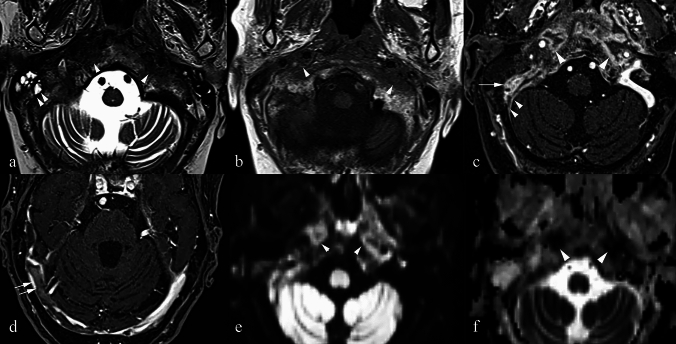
Elderly patient presenting with VII cranial nerve palsy and right-sided otalgia. a Axial T2 TSE at the level of the clivus and petrous apex demonstrates heterogeneous, low signal intensity changes throughout the bone marrow (arrowheads) associated with bilateral mastoiditis worse on the right (double arrowhead). b Axial T1 SE at the same level confirms the pathological marrow replacement c T1 VIBE post-contrast depicts irregular peripheral rim enhancement within the clivus and both petrous apices (single arrowheads) and lack of contrast filling of the right sigmoid sinus in keeping with thrombosis (arrow). Dural thickening and enhancement of the tentorium cerebelli is also appreciable on the ipsilateral side just beneath the thrombosed sigmoid sinus (double arrowhead). d Axial T1 VIBE post-contrast at the level of the transverse sinus reveals an extensive filling defect on the right in keeping with propagation of the thrombus beyond the sigmoid sinus described earlier in (c) (double arrow). e Axial DWI B1000 at the level of the petrous apex shows bilateral high signal which corresponds to low values on the ADC Map (f) confirming restricted diffusion (arrowheads)
